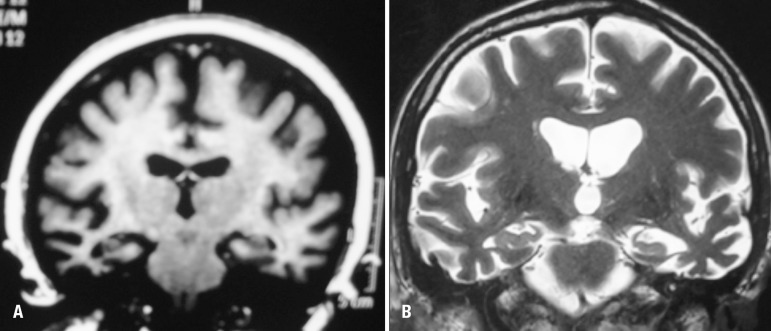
Examples of structural imaging (MRI) of cases 1 and 3.
